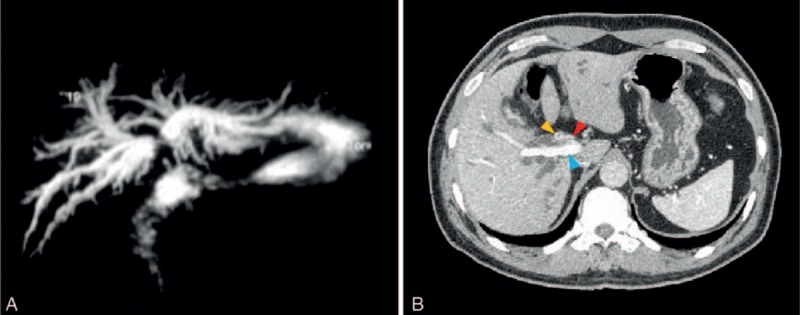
Imaging results for a 58-year-old man who presented with yellowish skin and sclera, abdominal distension, pruritus, and anorexia for 3 weeks. (A) Magnetic resonance cholangiopancreatography and (B) enhanced computed tomography showed a hilar cholangiocarcinoma (red arrow: right hepatic artery; yellow arrow: tumor; blue arrow: right portal vein).
